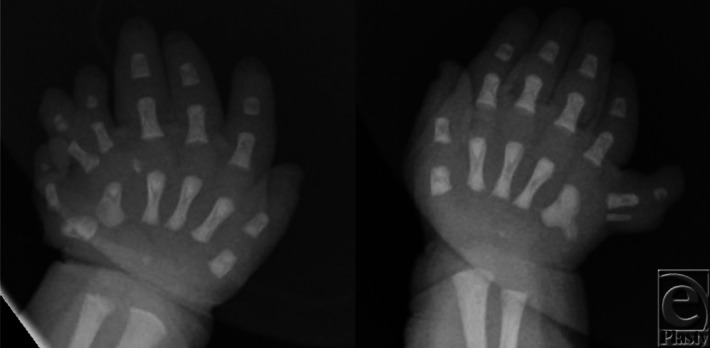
Anteroposterior x-ray images of the same patient.
